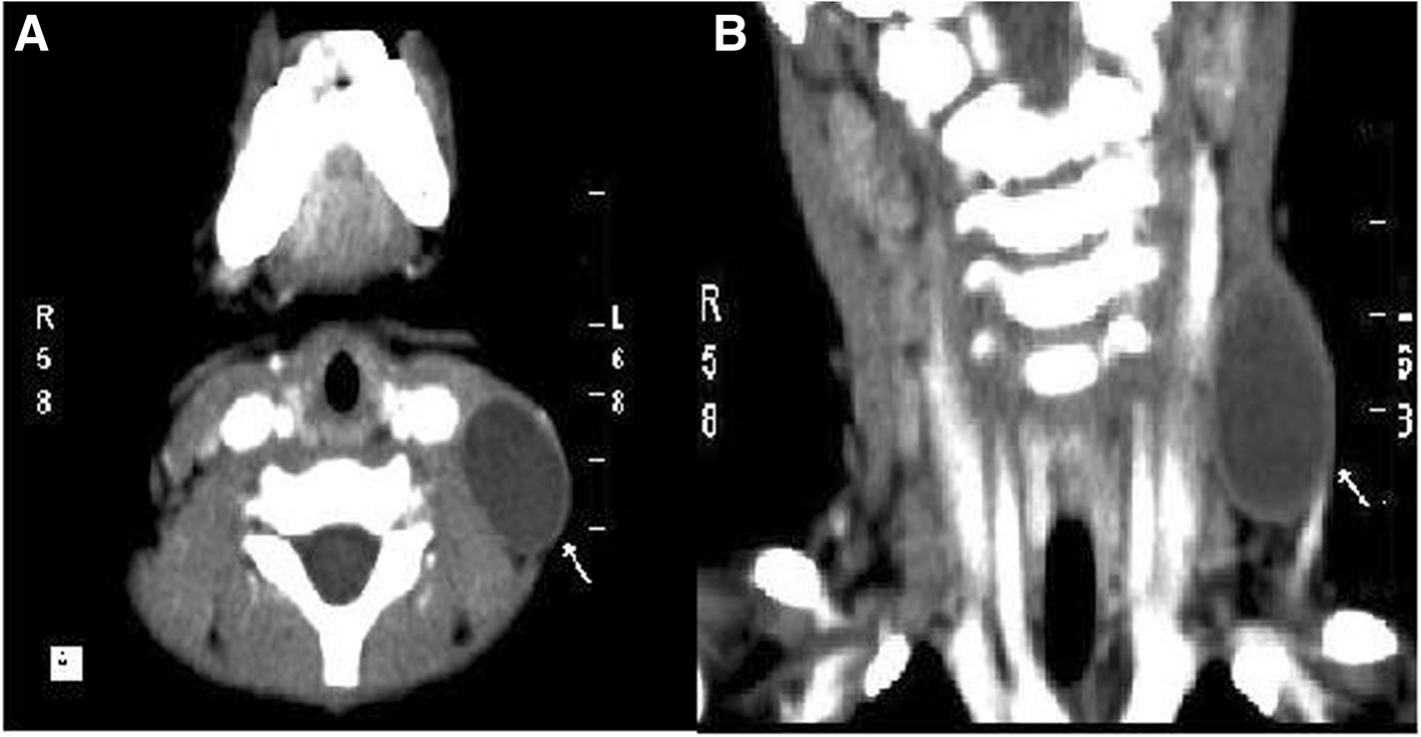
Neck computed tomography scan with contrast enhancement. a axial scan and b coronal scan; there is a 3 cm × 3 cm sized, well-encapsulated homogenous low-density mass in the left posterior triangle neck area (*arrows*)
